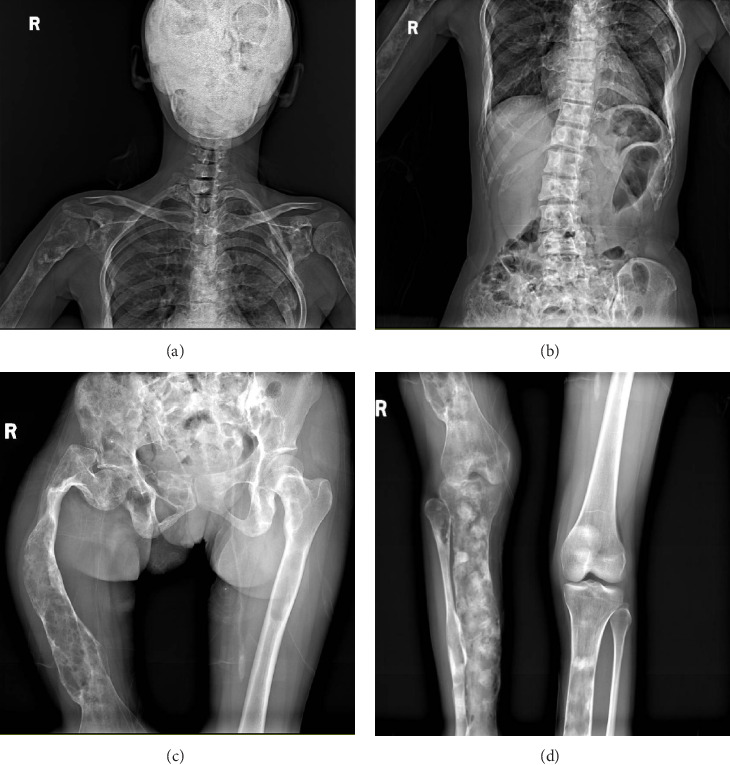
Radiographic examination at presentation revealed: (A) multiple osteolytic lesions in the bilateral ribs, scapulae, and humeri, (B) scoliosis with osteolytic lesions in the thoracolumbar spine, (C) classic “Shepherd's crook” deformity in the right proximal femur with osteolytic lesions in bilateral femora, and (D) osteolytic lesions in the bilateral tibiae and right fibula.
